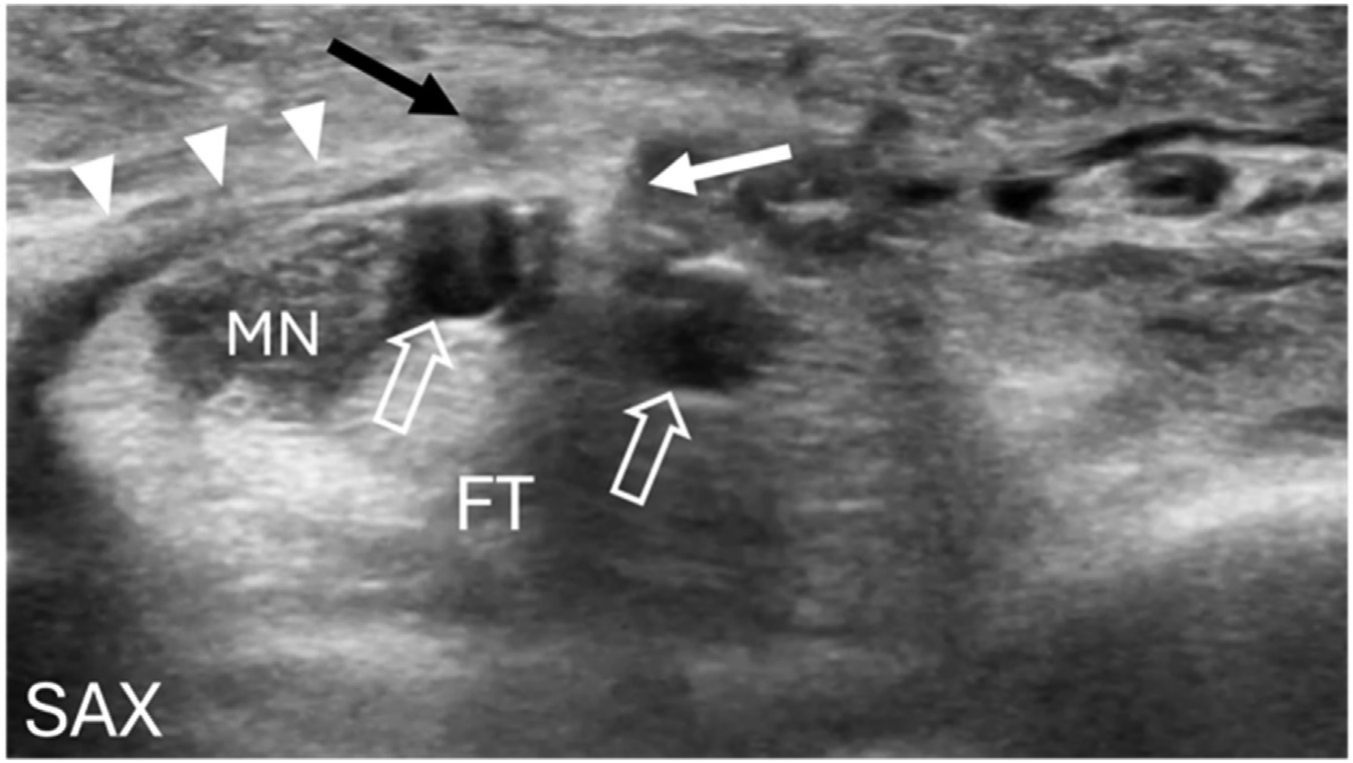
Transection of the transverse carpal ligament adjacent to the TLB of the MN. Short-axis or anatomic transverse US image during ultrasound-guided CTR. The device has been positioned in the carpal tunnel, ulnar to the MN. Following balloon deployment (open arrows) and confirmation of accurate positioning relative to the surrounding structures, the retrograde cutting blade (white arrow) has been activated from its recessed position. The cutting blade is shown adjacent and ulnar to the TLB (black arrow) during the retrograde cut of the TCL complex (arrowheads). FT = flexor tendons. Left = radial, Top = superficial.
